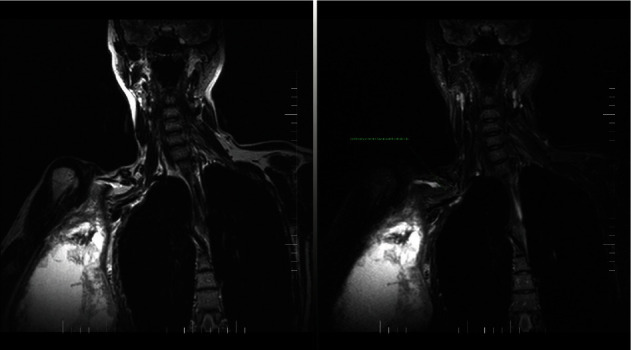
Magnetic resonance image (MRI T2 WI tse cor, STIR cor) shows the brachial plexus and its branches with a pathological signal. The MRI scan corresponds with the last recurrence of the tumor.
